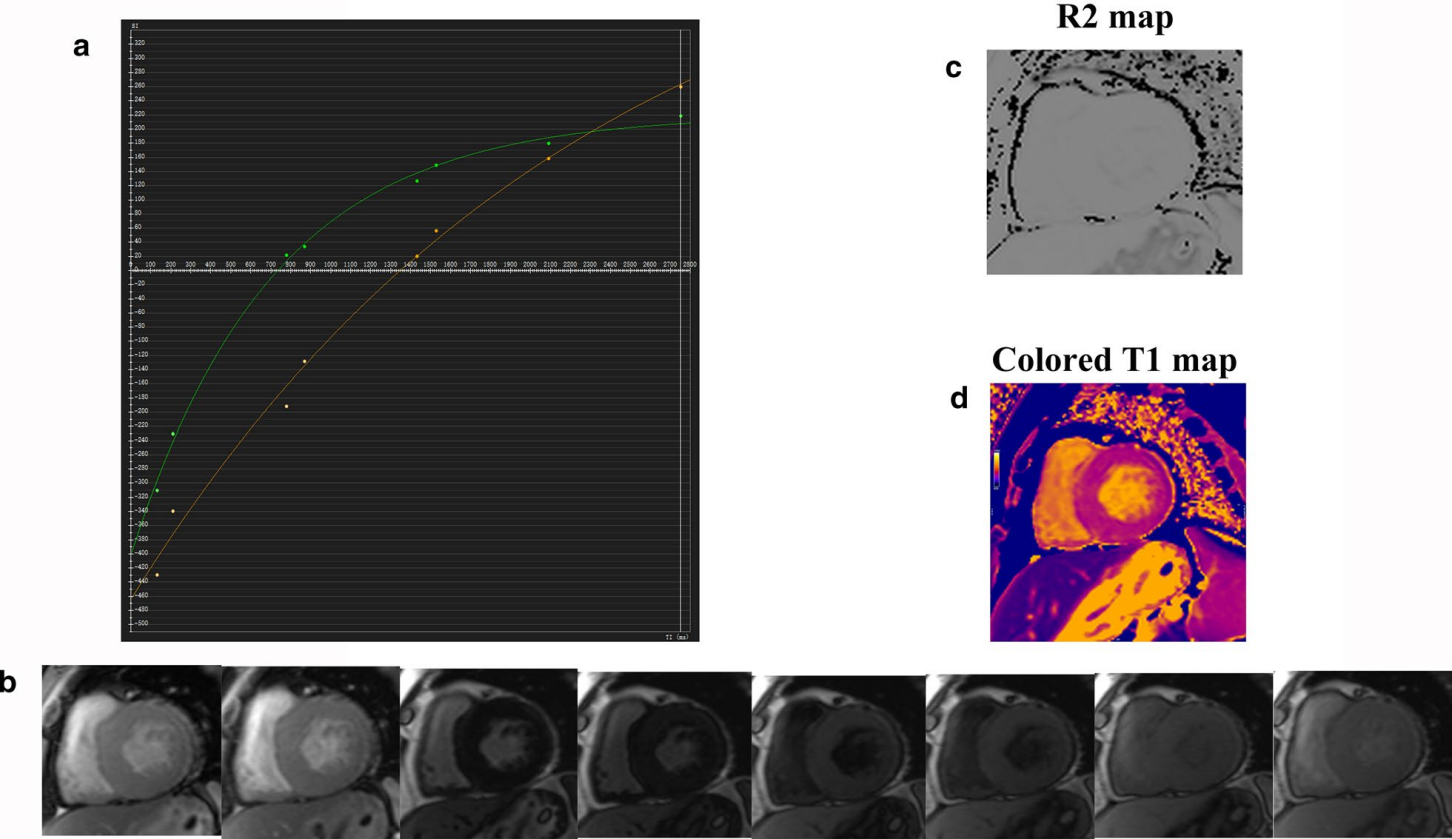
Inversion recovery curves of T1 mapping. The inversion recovery curve (a) and R2 map are generated from the T1-weighted source images (b). The signal intensity in each pixel of T1-weighted source images is used to fit the colored T1 (d) and R2 maps (c) that are generated
